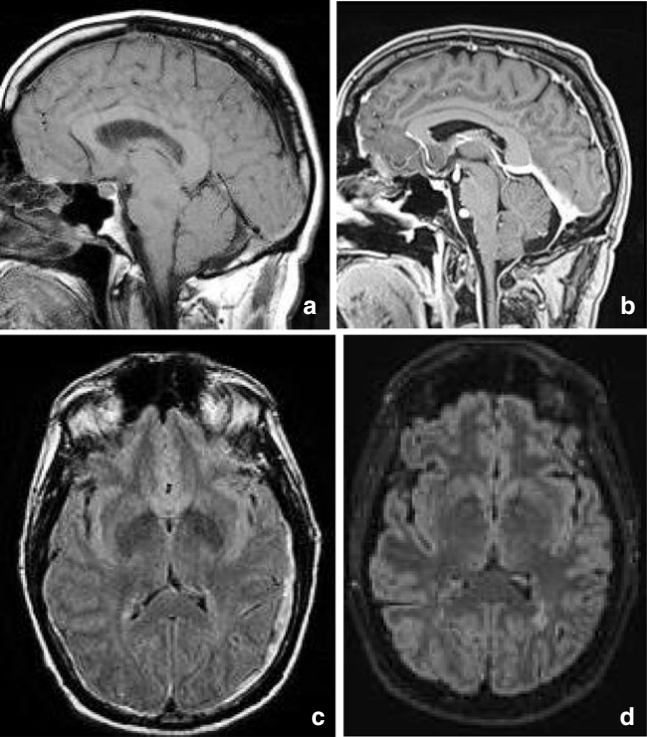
Fifty-three-year-old man with intracranial hypotension syndrome. At first admission, sagittal T1 (a) shows an enlarged and downward herniating splenium, with normal signal intensity on the axial FLAIR (c). Also sagging midbrain and bilateral subdural hematoma. Seven years later scanned for left frontal headache, disappearing with reclining. Sagittal T1 after gadolinium (b) shows normalized position brainstem, with decreased enlargement of the splenium. On axial FLAIR (d), no new SDH is seen
